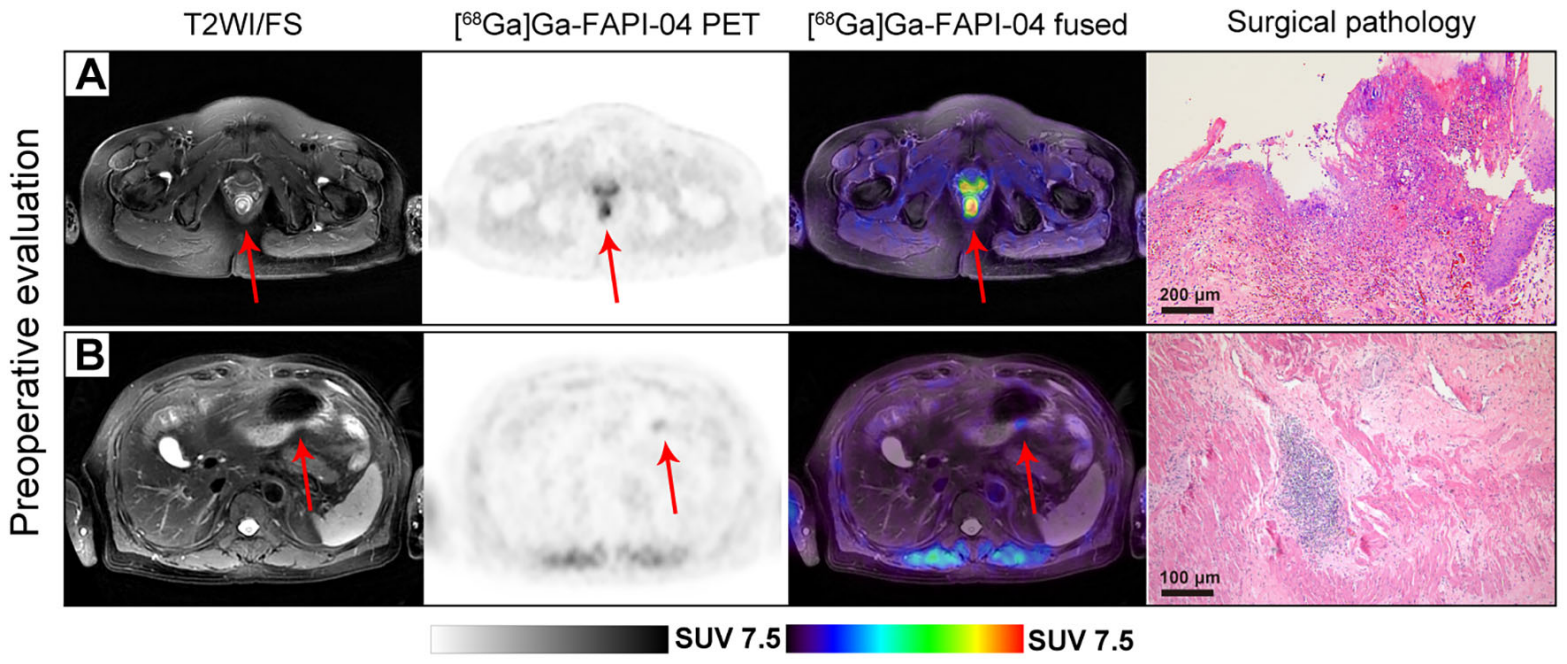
(A) A patient in her 50s presented with a 2-month history of hematochezia, which led to the diagnosis of adenocarcinoma in the lower rectum. Following neoadjuvant short-course radiotherapy combined with immunochemotherapy, [^68^Ga]Ga-FAPI-04 PET/MR revealed avid tracer accumulation (SUVmax 6.8, SULpeak 3.76, FAPI-PTV 1.41 cm^3^, FAPI-TL 7.56, indicated by red arrows) in the lower rectum. However, subsequent surgical pathology revealed no residual tumor cells (pCR), although a chronic ulcer with hyperplasia of granulation tissue was observed. (B) In another case, a 75-year-old man underwent chemotherapy for gastric adenocarcinoma. A slight focal uptake (SUVmax 2.18, SULpeak 1.75, FAPI-PTV 1.87 cm^3^, FAPI-TL 2.35) was observed in [^68^Ga]Ga-FAPI-04 PET/MR imaging, but further surgical pathology confirmed the lesion as pCR.
